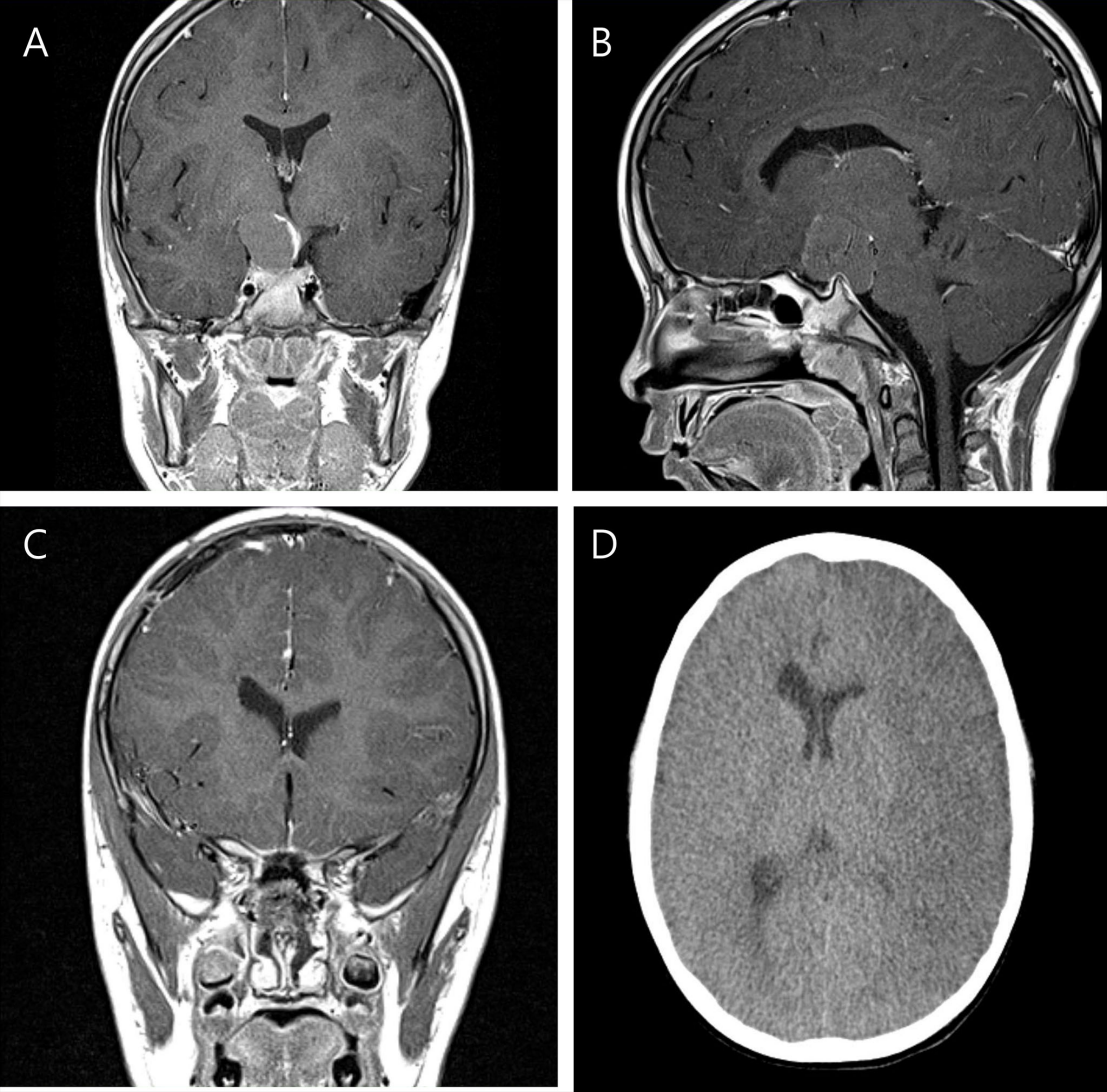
Preoperative T-1 enhanced coronal (A) and sagittal (B) images of a six-year-old boy with craniopharyngioma. Postoperative MRI taken within 48 hours shows successful removal of the tumor without any abnormal finding (C). However on postoperative day 6 after rapid fluctuation of his serum sodium level, low attenuation along the MCA territory was found (D), suggestive of cerebral infarction.
